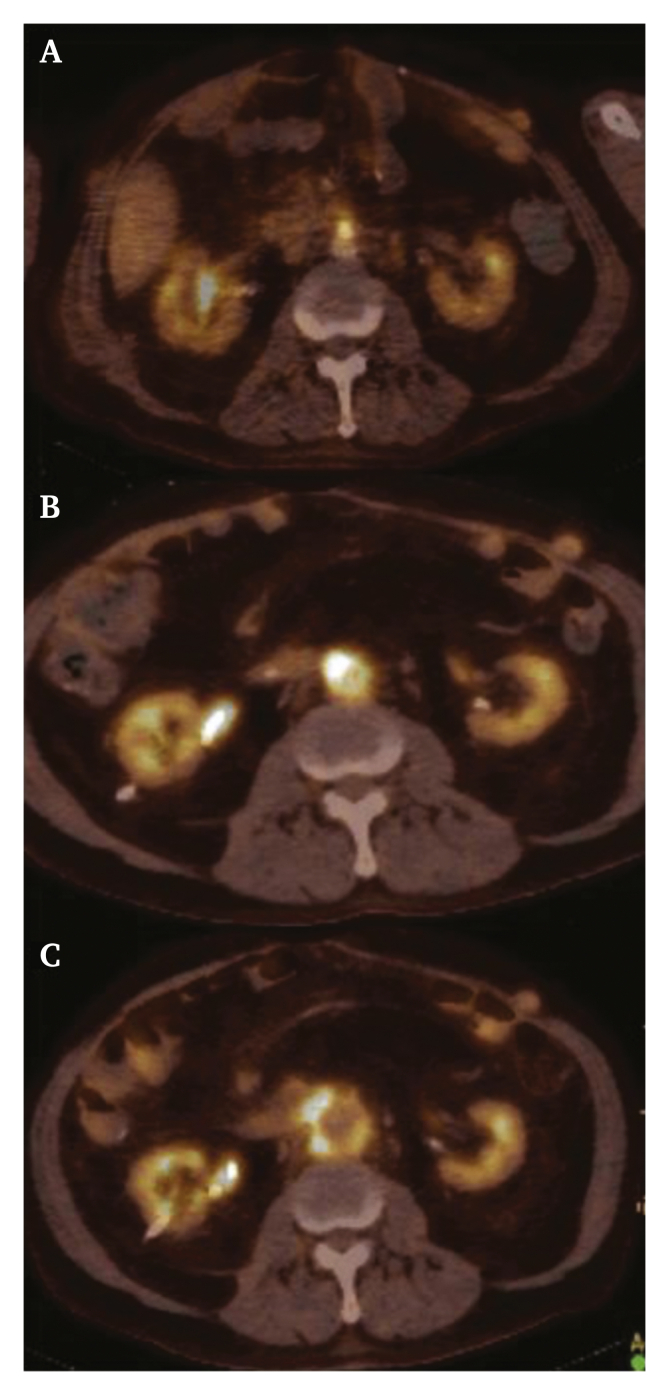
PET scan demonstrating persistent infection and progressive dilatation of the aortic stump. PET-scans were performed which demonstrated persistent infection of the aortic stump, with the Amplatzer® Vascular Plug in situ. Furthermore, it showed progressive dilatation of the aortic stump without signs of acute rupture. The upper image (A) shows small amounts of fluor-18-glucose (FDG) uptake around the aortic stump (diameter 20mm) in 2015 (two years after the endovascular repair). The middle image (B) shows increased FDG uptake and signs of enlargement of the aortic stump (diameter 23mm) at the beginning of January 2019. The lower image (C) demonstrates further increase in FDG uptake around the aortic stump and progressive enlargement (diameter 36mm) by the end of January 2019.
